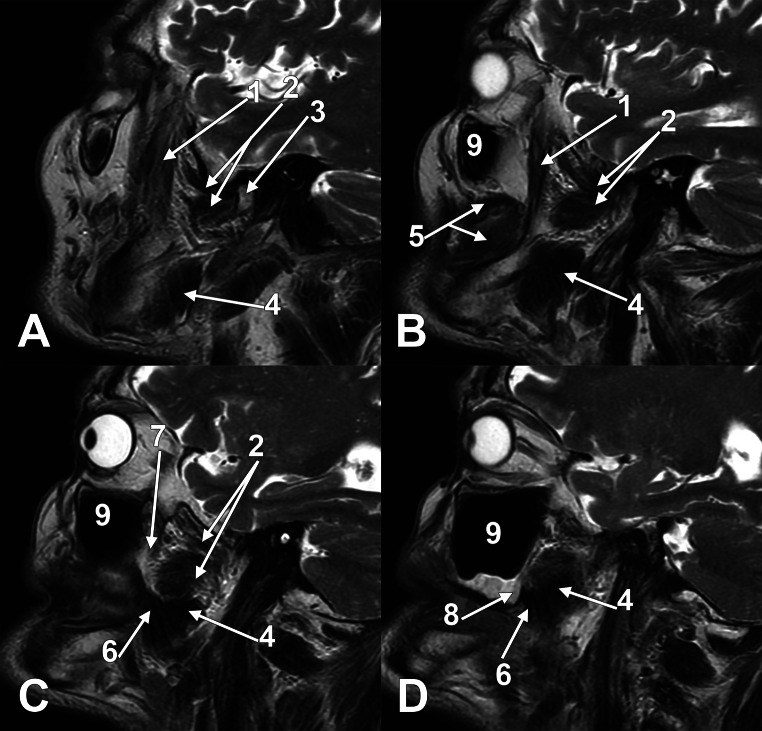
Lateral-to-medial series of sagittal MRI slices through the masticatory muscles. The medial pterygoid muscle’s superficial (accessory) head is attached to the distal maxillary alveolar bone. (1) temporal muscle (anterior bundle); (2) lateral pterygoid muscle; (3) head of the mandible; (4) deep (main) part of the medial pterygoid muscle; (5) buccinator muscle; (6) superficial (accessory) head of the medial pterygoid muscle; (7) posterior (infratemporal) face of the maxillary body; (8) distal end of the alveolar process of maxilla; (9) maxillary sinus
